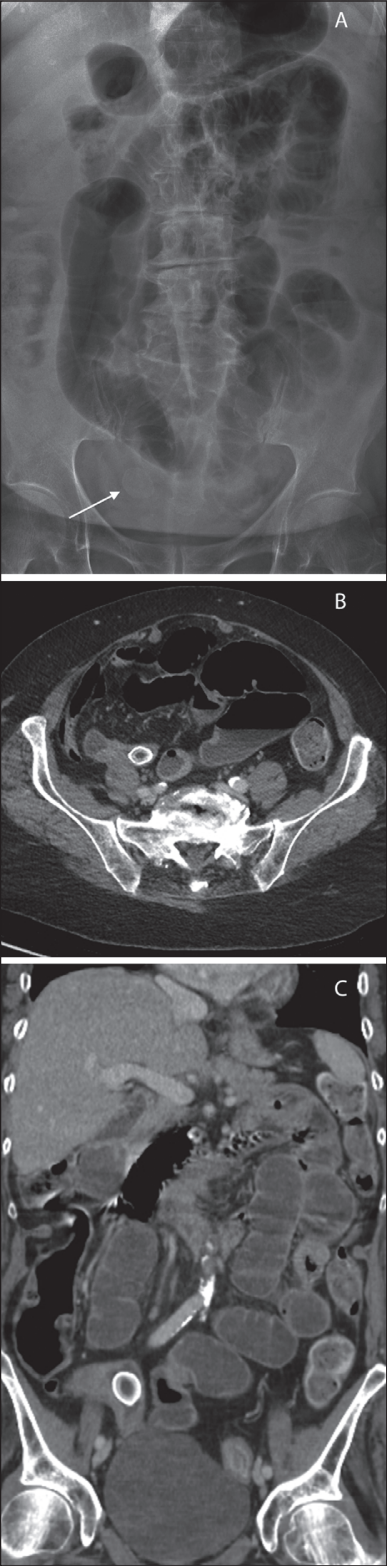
A small bowel obstruction caused by a corpus alienum was diagnosed on the basis of radiograph, ultrasound and CT findings.
